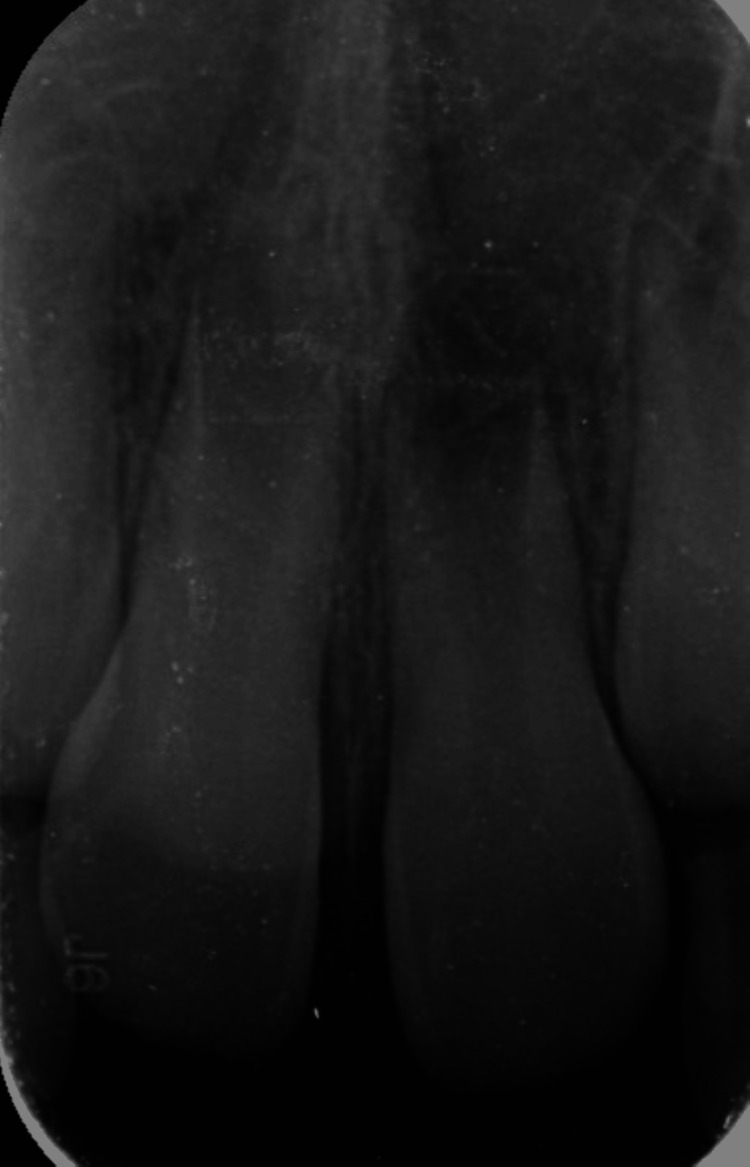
Digital radiograph (radiovisiography) confirming the presence of the embedded central incisors (11, 21)
